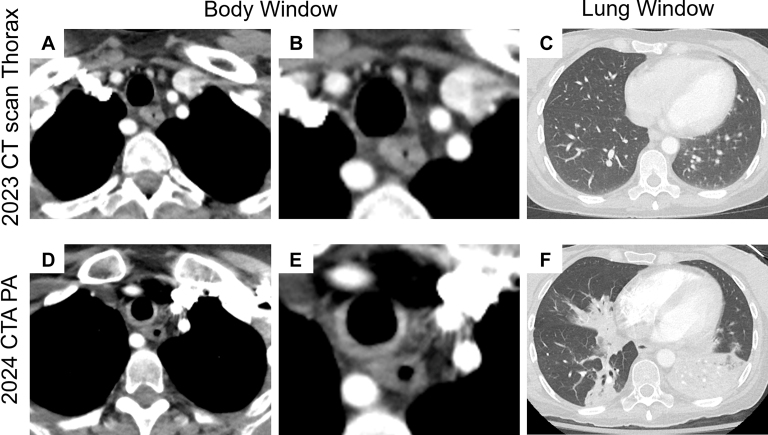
A-F, Chest CTA with pulmonary artery protocol on admission (D, E) demonstrating circumferential upper airway wall thickening and soft tissue density that extends along the bilateral proximal airways. Lung windows of the same scan show consolidative opacities most notable in the left lower lobe but also within the medial aspect of the right middle lobe and right lower lobe (F). CT thorax scan from 1 y before admission provided for reference with corresponding cuts (A-C). CTA = CT angiography; PA = pulmonary artery.
